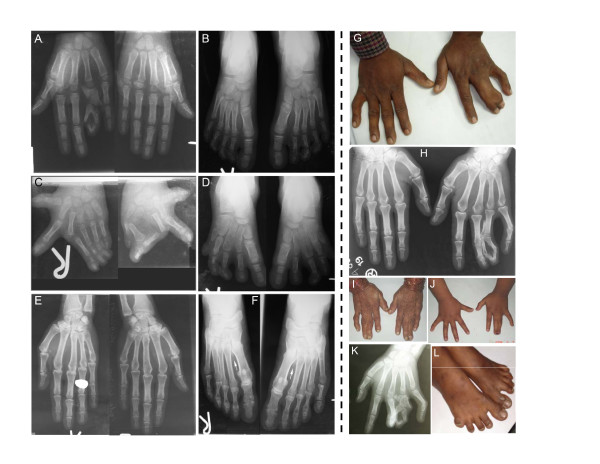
SPD1 phenotype in the Asian families. Radiographs and photographs showing the SPD1 phenotype in the Pakistani (A – F), and Indian (G – K) families. A-B, (subject IX-23); C-D, (IX-20); E-F, (VIII-24); G-H, (IV-7); I, (III-3); J, (V-6); K-L, (V-3). Phenotypic categories are given in Figure 3.
